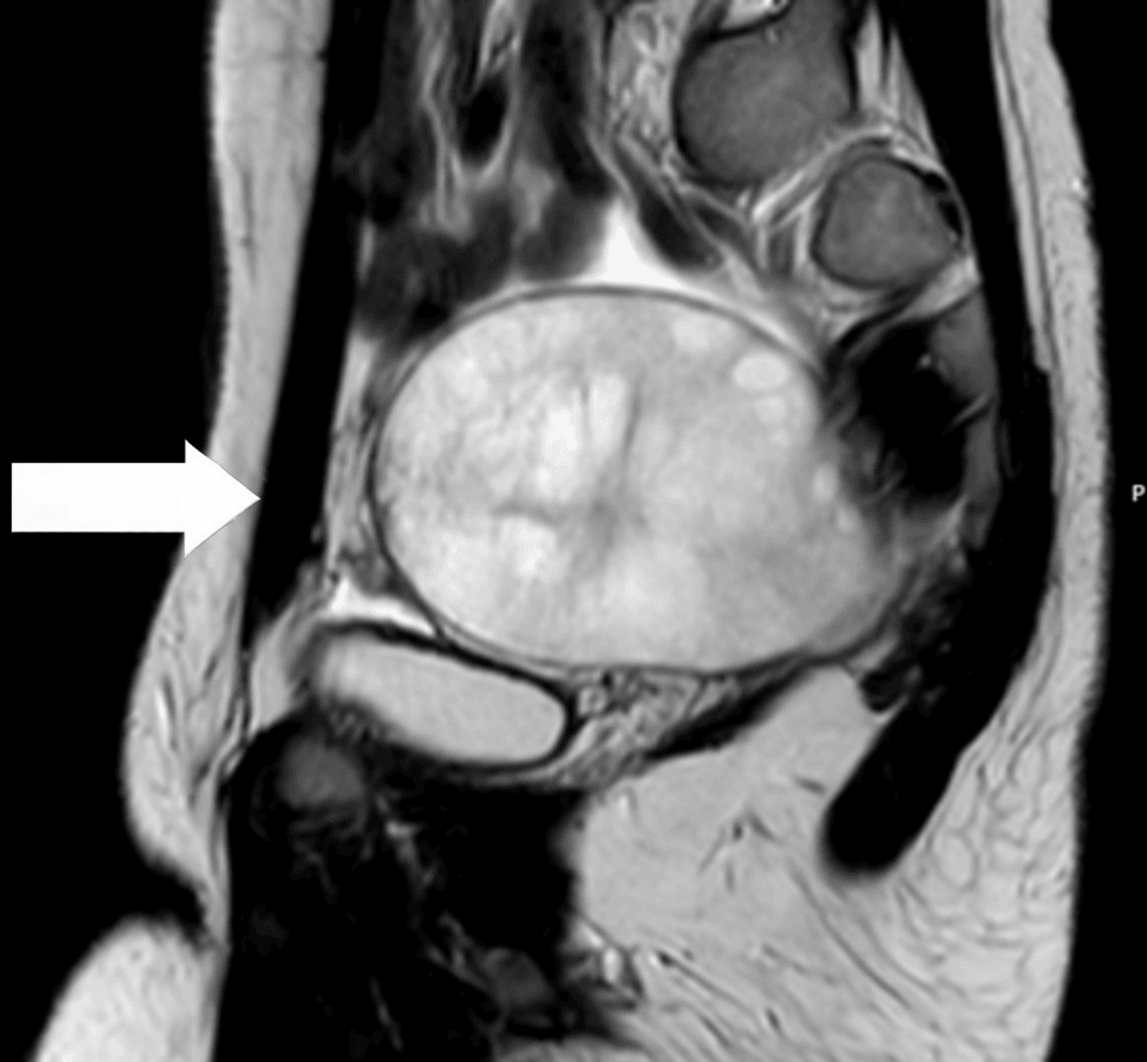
Pelvic MRI showing adnexal mass in sagittal section
